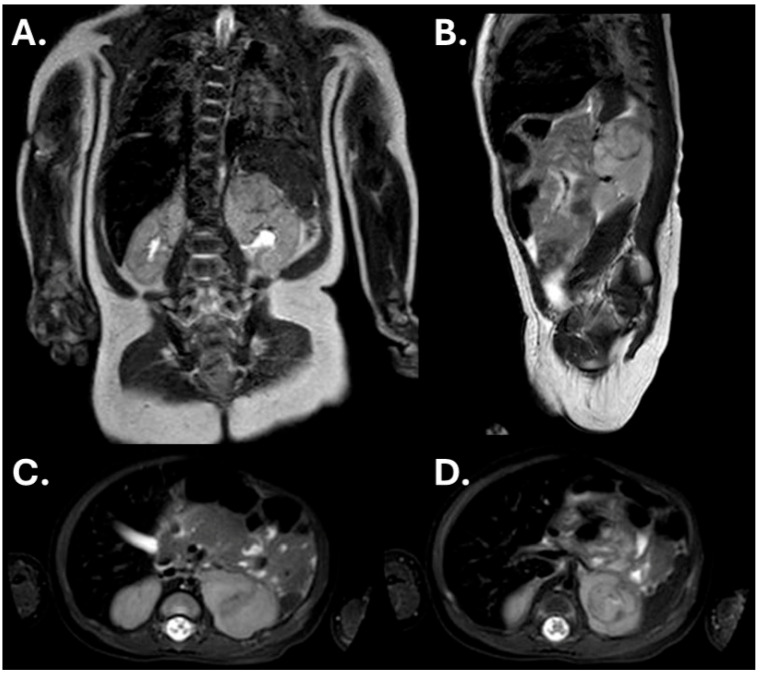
MRI of the abdomen at diagnosis. Coronal (A) and sagittal (B) T2-weighted images demonstrate a well-circumscribed solid mass centered in the upper pole of the left kidney. Axial T2-weighted fat-suppressed images (C,D) show a predominantly homogeneous solid tumor.
